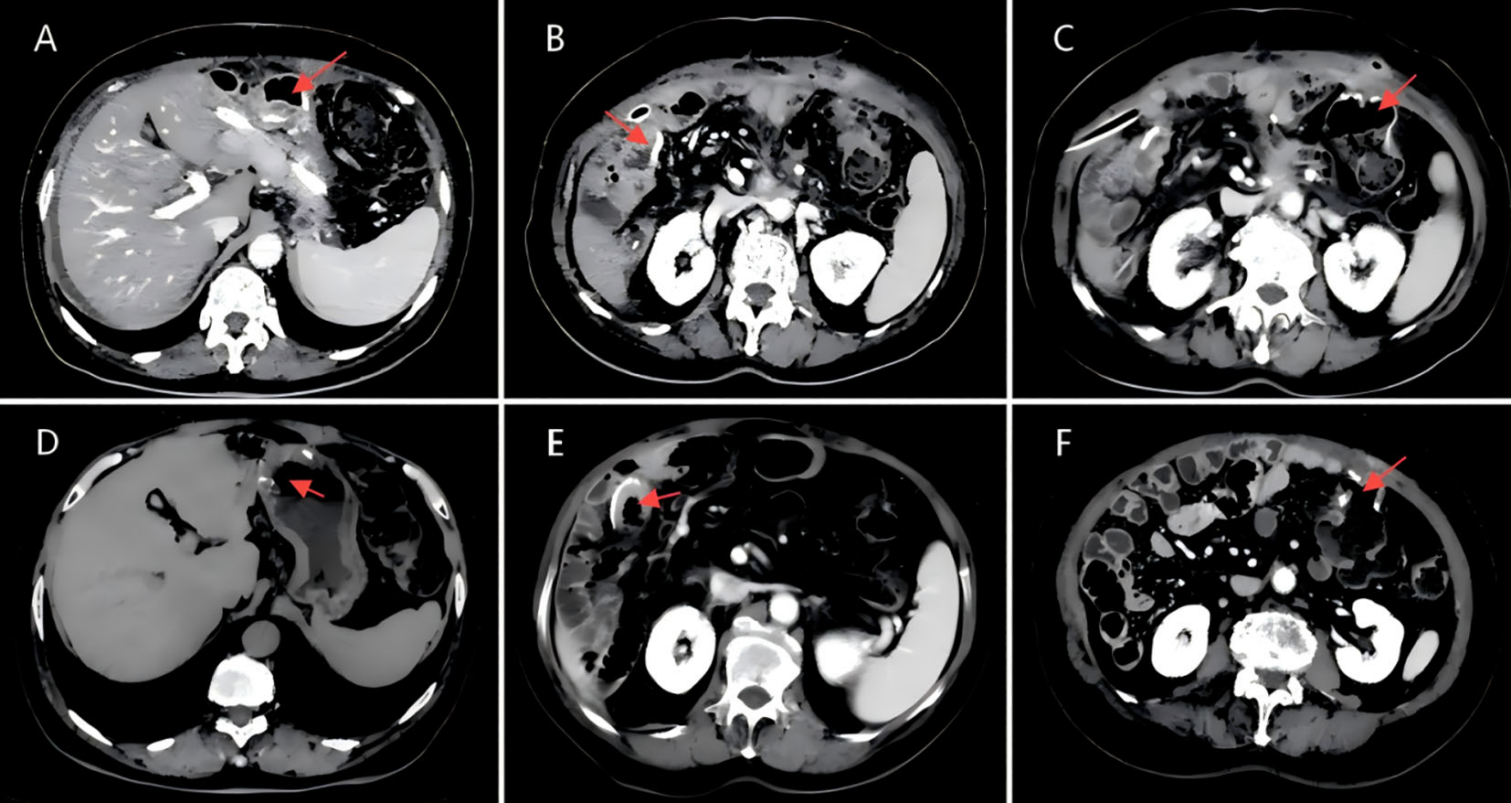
(A)CT scan image of the gastro-intestinal anastomosis, dated March 19, 2024.(B)CT scan image of the biliary-enteric anastomosis, dated March 19, 2024.(C)CT scan image of the ileocolic anastomosis (ileum to transverse colon), dated March 19, 2024.(D)CT scan image of the gastro-intestinal anastomosis, dated November 26, 2024.(E)CT scan image of the biliary-enteric anastomosis, dated November 26, 2024.(F)CT scan image of the ileocolic anastomosis (ileum to transverse colon), dated November 26, 2024.
